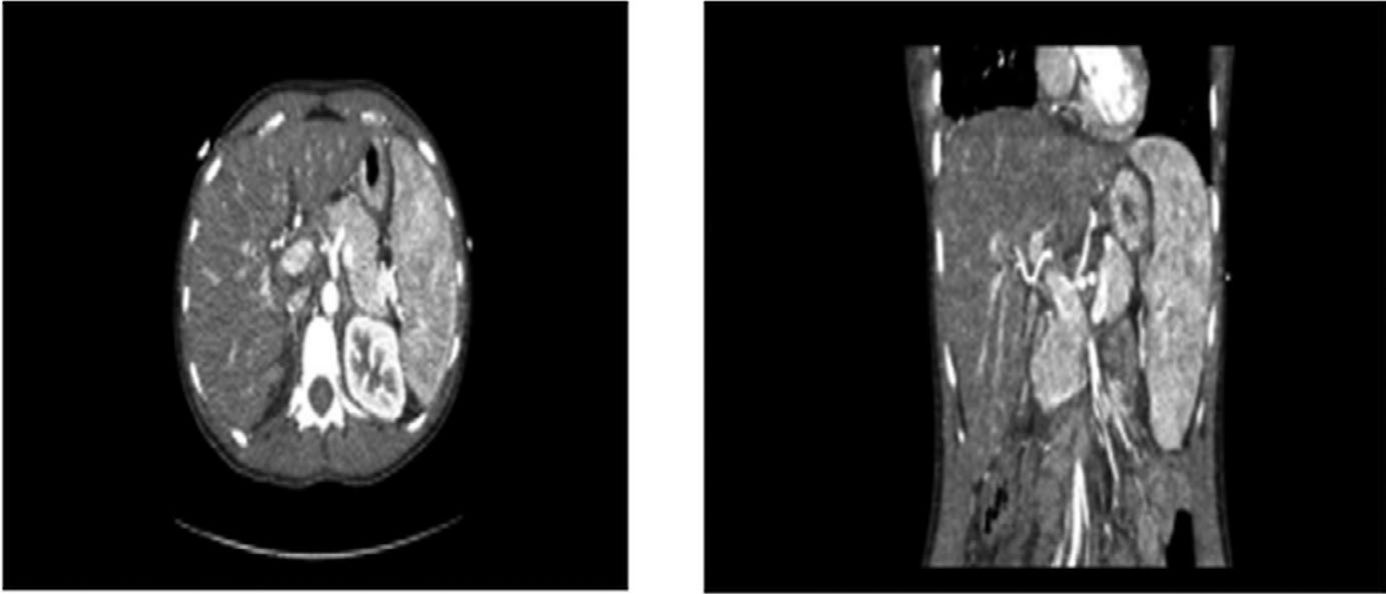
CT Abdomen: Lymphadenopathy with marked hepatosplenomegaly
